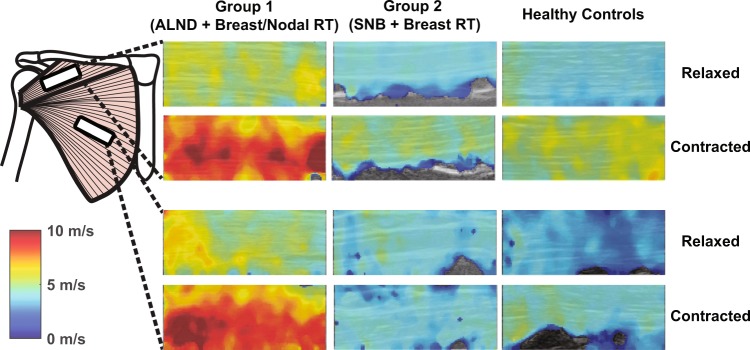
Representative ultrasound shear wave elastography maps collected from one breast + nodal RT patient (Group 1 – 1^st^ column), one breast RT only patient (Group 2 – 2^nd^ column), and one age-matched healthy control (3^rd^ column). Ultrasound images were collected from both the clavicular region (top six images) and sternocostal region (bottom six images) of the pectoralis major. In each region, the muscle was examined both in a relaxed state (top row) and contracted state (bottom row). The muscle contraction displayed here was scaled to 10% of the participant’s strength in horizontal flexion. A color scale bar is shown in the lower-left corner, indicating the measured shear wave velocity in the elastography color maps. A brighter color is indicative of a stiffer tissue.
